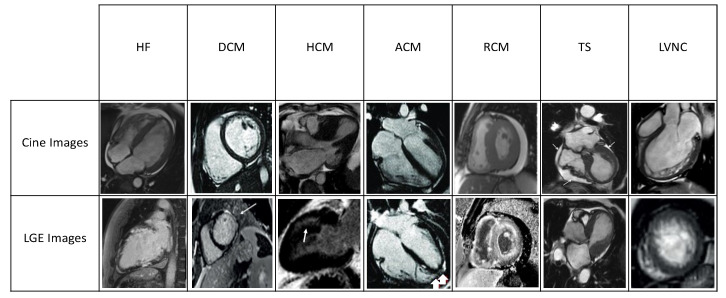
Cardiomyopathies seen through cardiac MRI. HF: heart failure; DCM: dilated cardiomyopathy; HCM: hypertrophic cardiomyopathy; ACM: arrhythmogenic cardiomyopathy; RCM: restrictive cardiomyopathy; TS: Takotsubo syndrome; LVNC: left ventricular noncompaction.
